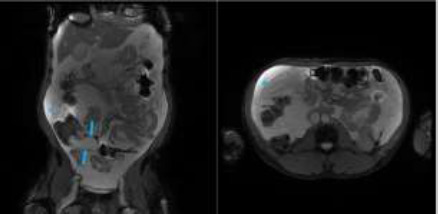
Coronal axial T2 weighted with fat suppression Magnetic Resonance Images of the abdomen and pelvis showing fluid-filled tubular dilatation of the appendix (long arrows), with associated infiltration of the omentum (short arrows) and gross ascites
